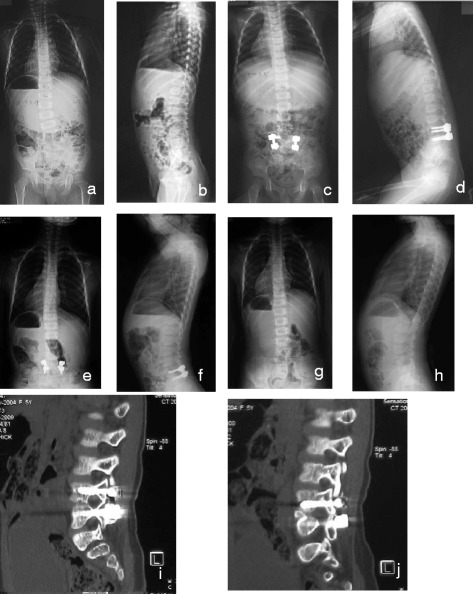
An infant with congenital scoliosis. Preoperative radiographs (a, b) showed fully segmented hemivertebra of L5/6 with the Cobb angle of 27°. Postoperative radiographs (c, d) showed excellent correction by L5/6 hemivertebra resection with the Cobb angle of 2°. Radiographs at 3 years follow-up (e, f) showed excellent correction with the Cobb angle of 4°. CT scan (i, j) showed solid fusion at the fused segments and L5 right pedicle screw dislodgement. And implants were removed (g, h)
